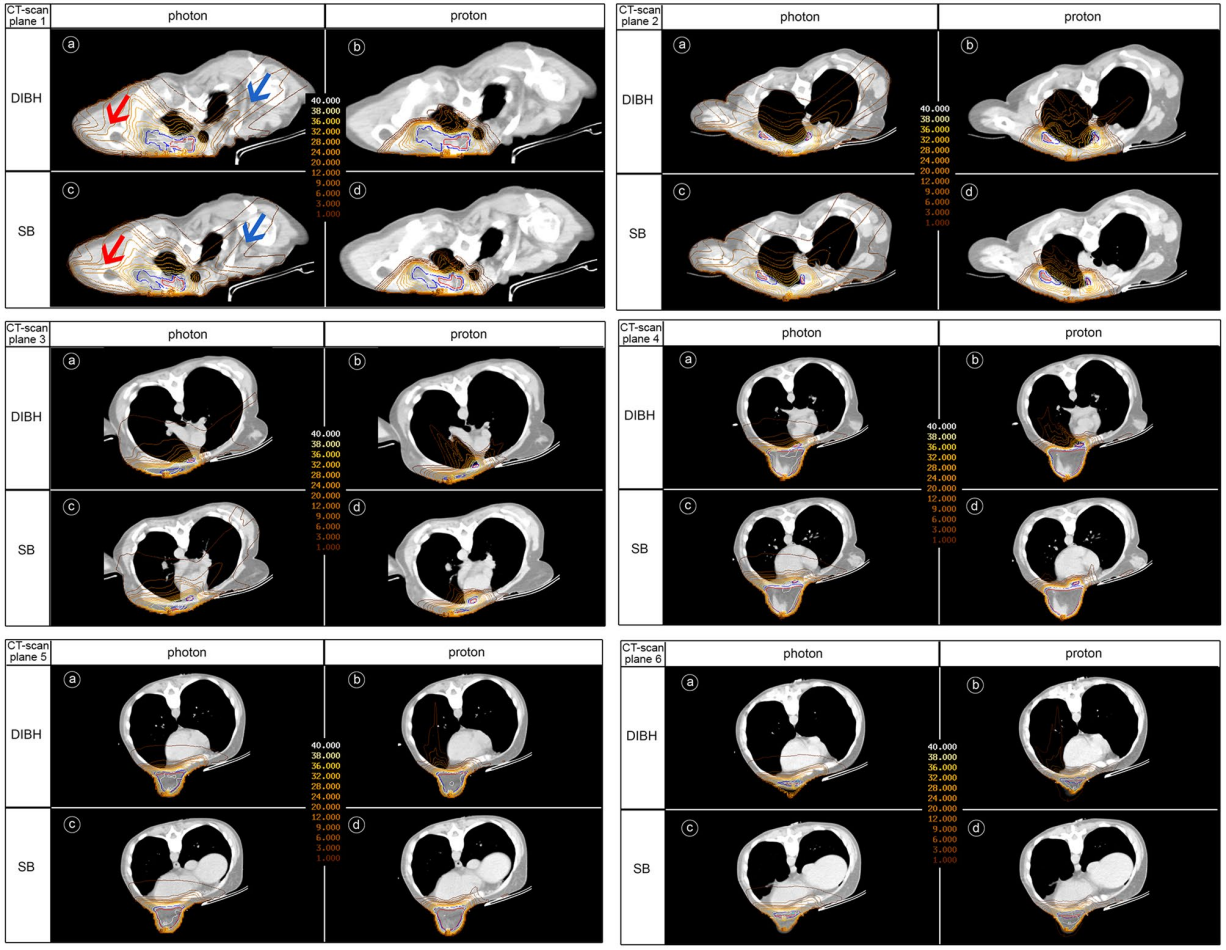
Transverse dose distribution differences between DIBH and SB photon and proton plans. For photon VMAT DIBH and SB (CT scan planes 1–6: a,c) plans, a large dose spread is seen outside the target volumes in the dorsal shoulder region and inside the upper thorax^13^. For IMPT proton plans, a larger dose spread is clearly observed in DIBH where tissues of high density were replaced by tissues of low density (CT scan planes 1–6: b,d). Plane 1: lung top. Plane 2: near the cranial edge of the left internal mammary lymph node chain. Plane 3: near the cranial edge of the left breast. Plane 4: near the caudal edge of the internal mammary lymph node chain at the central part of the left breast. Plane 5: through the caudal quadrants of the left breast. Plane 6: near the caudal edge of the left breast. CT scan planes 3–6 show in the SB setting (d) that distal edges of proton pencils are located in the heart, blood vessels and pericardial fat. These ‘high’ density tissues protect the lung in SB. In the DIBH proton setting (b), high density tissue is replaced by low density lung tissue resulting in increased proton range. Proton dose is deposited far outside the deep edge of the target into the left lung tissue in the DIBH plan (b) compared to SB plan (d).
